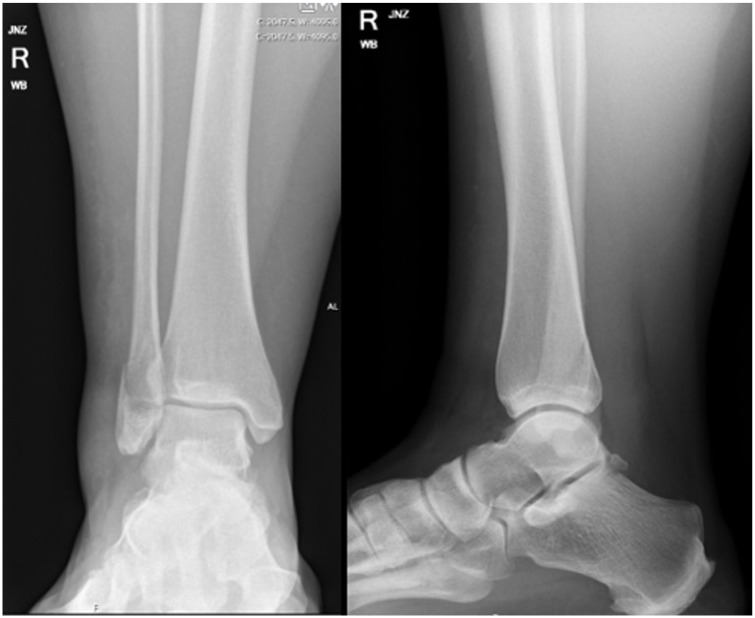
Anterior-posterior and lateral ankle weightbearing radiographs demonstrating a minimally displaced distal fibula fracture.
